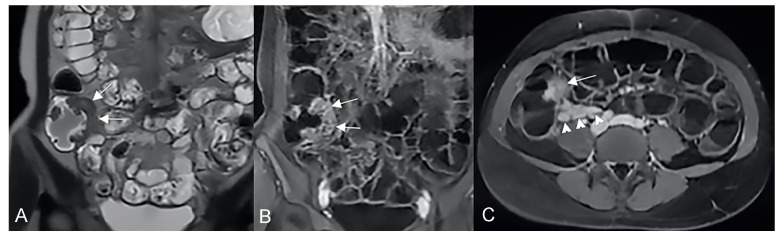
A case of ileal tuberculosis in a 34-year-old female patient. (A) Coronal T2-weighted image and (B,C) T1-weighted after gadolinium injection images in coronal and axial planes show thickening of the distal ileum and ileocecal valve (arrows). Enlarged lymph nodes are also observed in the adjacent mesentery (arrowheads).
